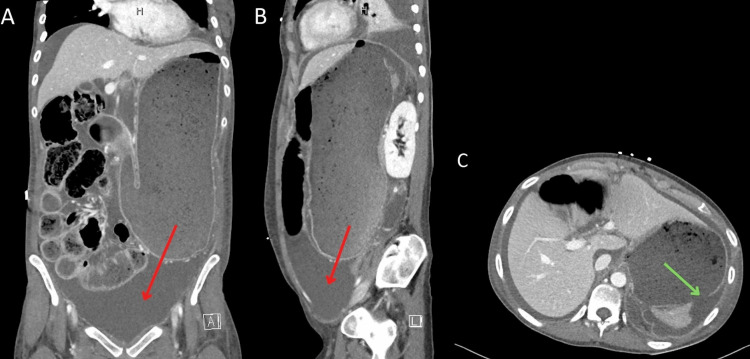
Coronal (A), sagittal (B), and axial (C) abdominal CT scans showing gastric perforation (green arrow) with intraperitoneal spillage of contents (red arrows).
